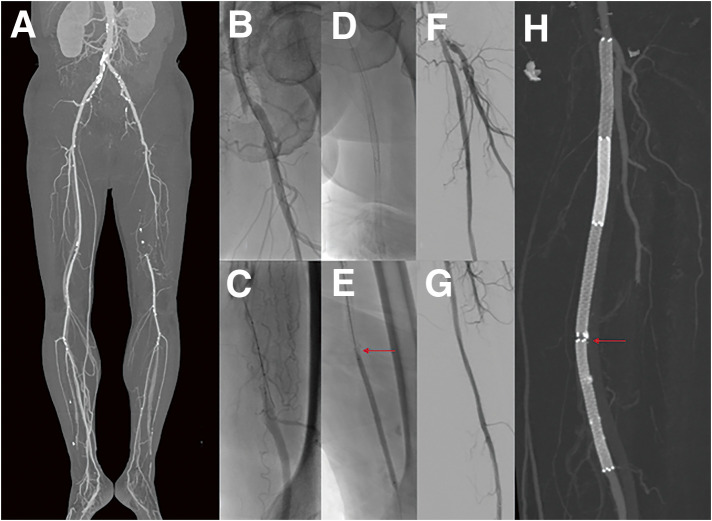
(A) Preoperative CT angiogram revealing a long SFA occlusion measuring 25 cm, with reconstitution distally at the proximal popliteal artery. (B) From a right-side up-and-over approach, the angiogram revealed proximal SFA occlusion with a visible orifice of SFA. (C) The antegrade approach failed to re-enter distally, necessitating retrograde puncture. (D) Proximal SFA: Eluvia stent (7 × 120 mm); mid-SFA: Eluvia stent (6 × 120 mm). (E) Short overlap (red arrow) between the mid-SFA stent and distal SFA stent (6 × 80 mm). (F–G) Final angiogram revealing good stent expansion and hemostasis of the retrograde puncture in the P1 segment. (H) Follow-up CTA at day 4 revealing stent migration causing a stent-free lesion (red arrow) between the distal 2 stents.
